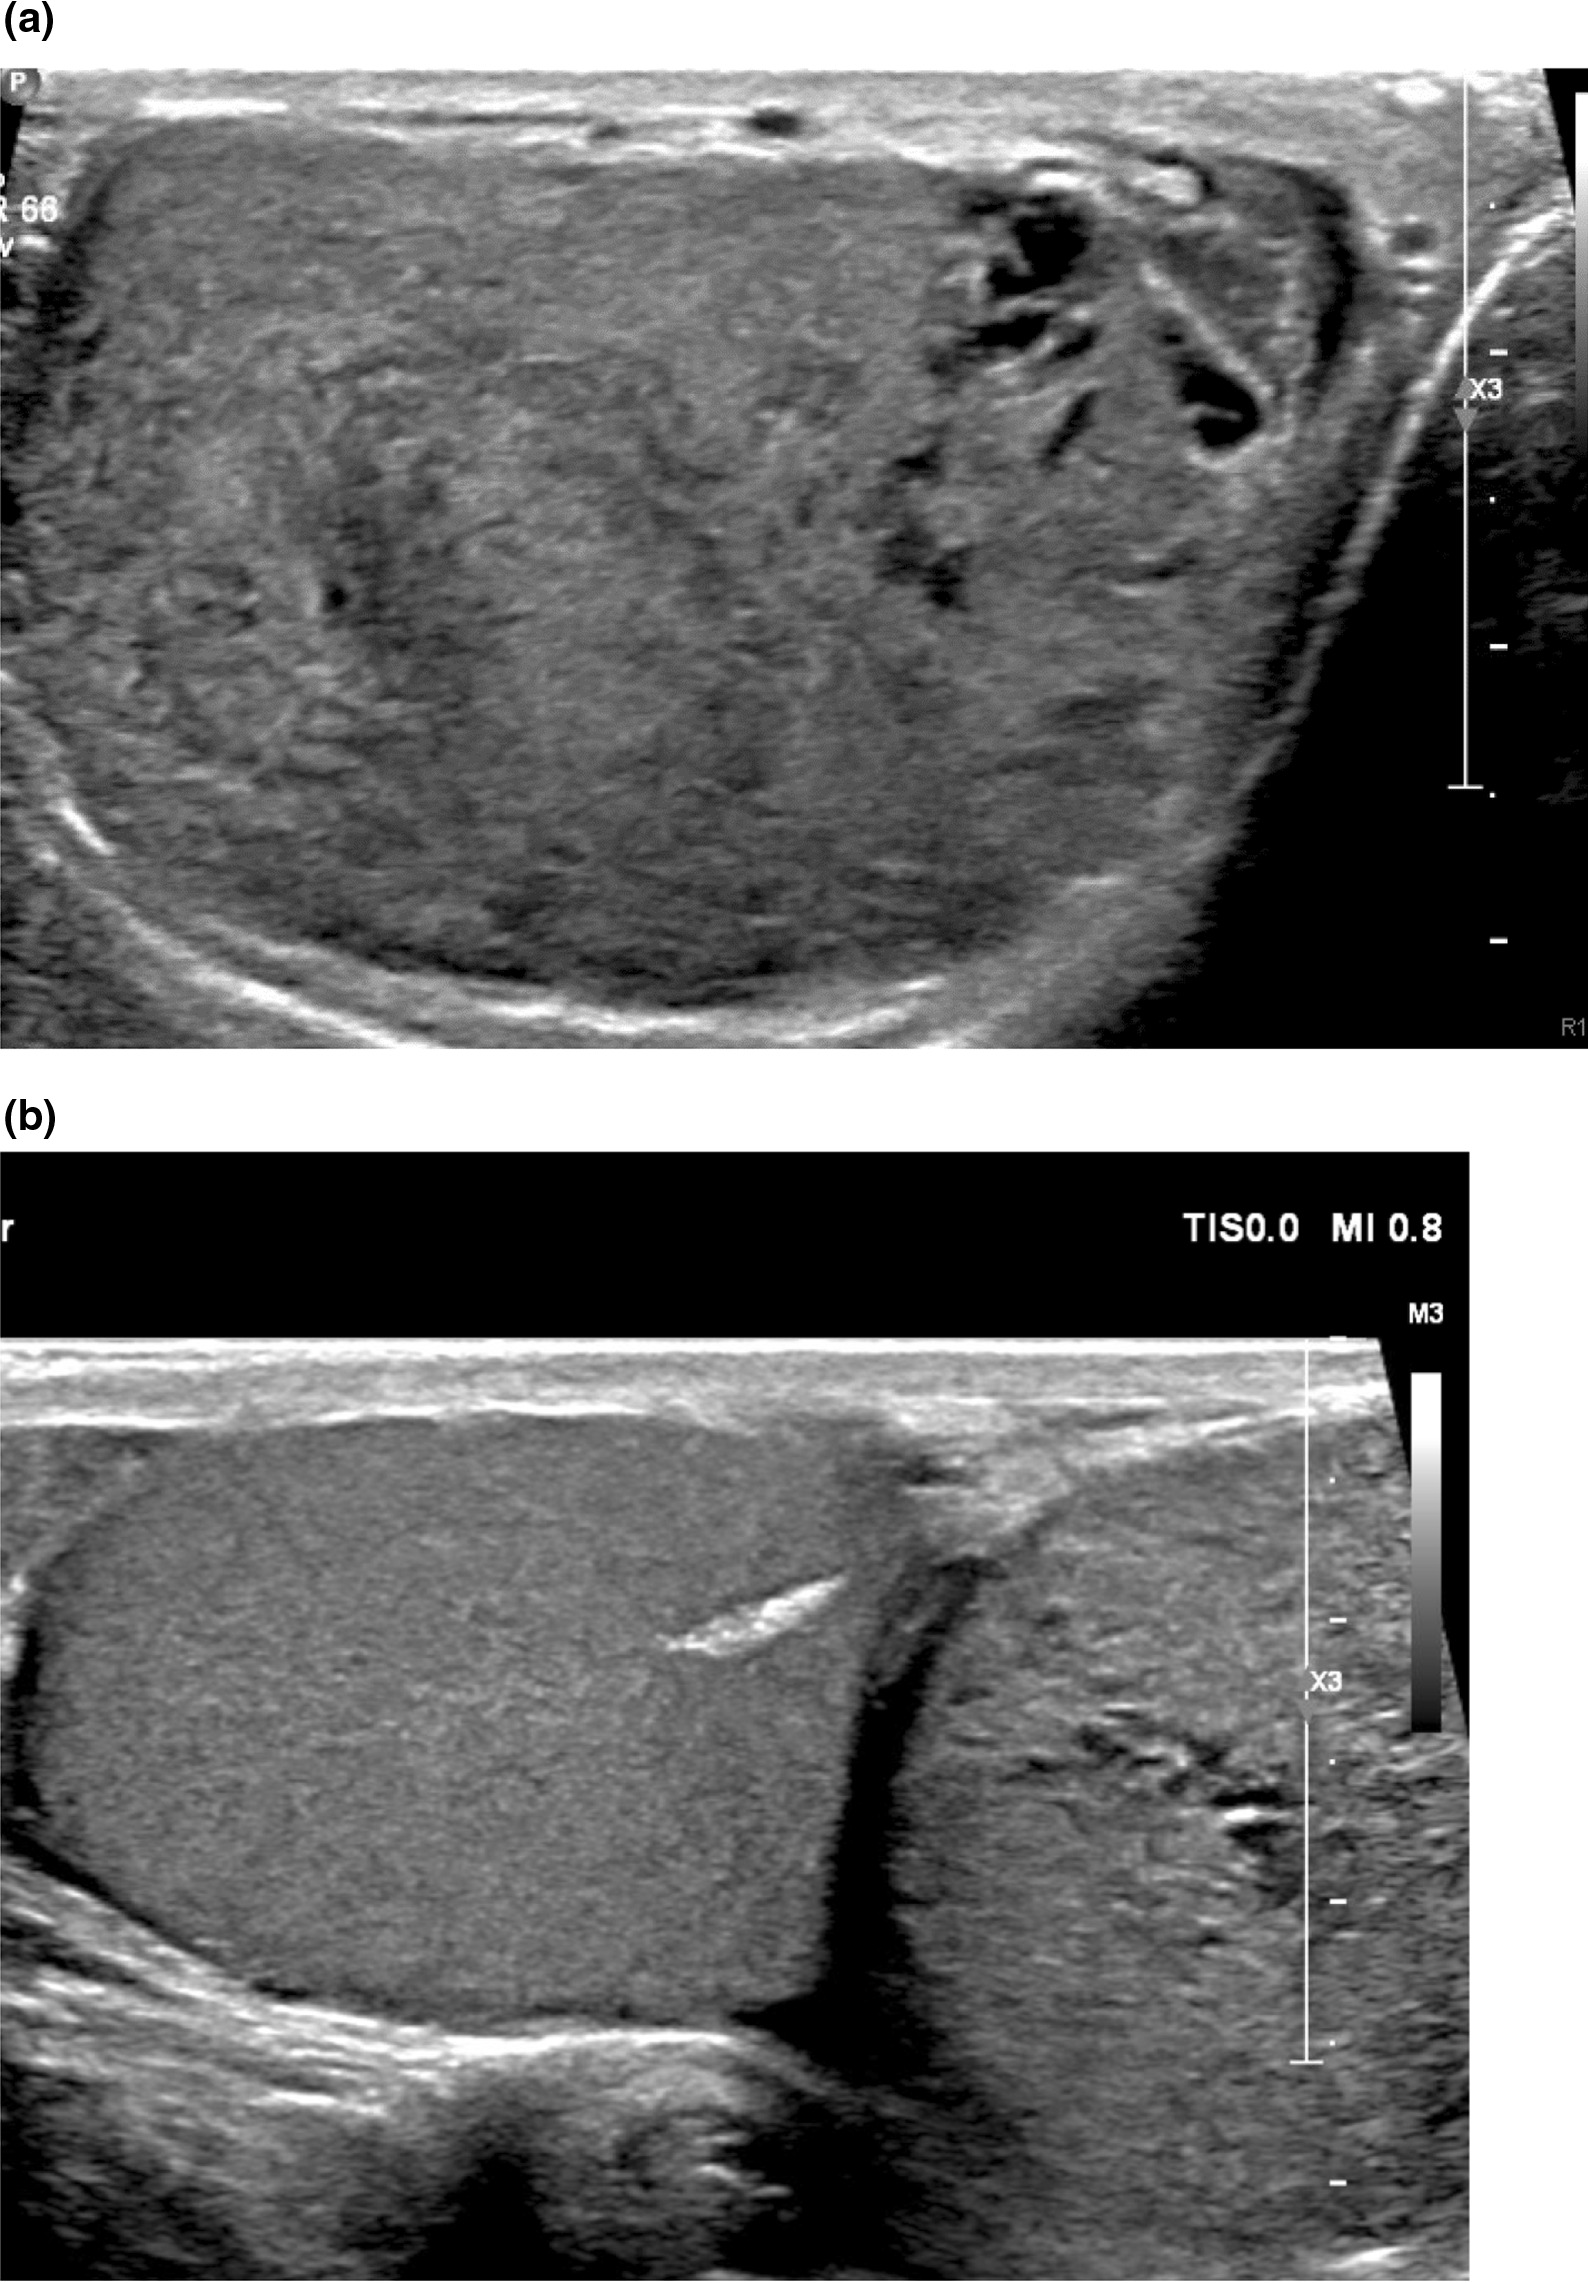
a (top image) Extratesticular mass on ultrasound in the right hemiscrotum, b (bottom image) side-by-side views of both testicle (left) and part of extratesticular mass (right)
